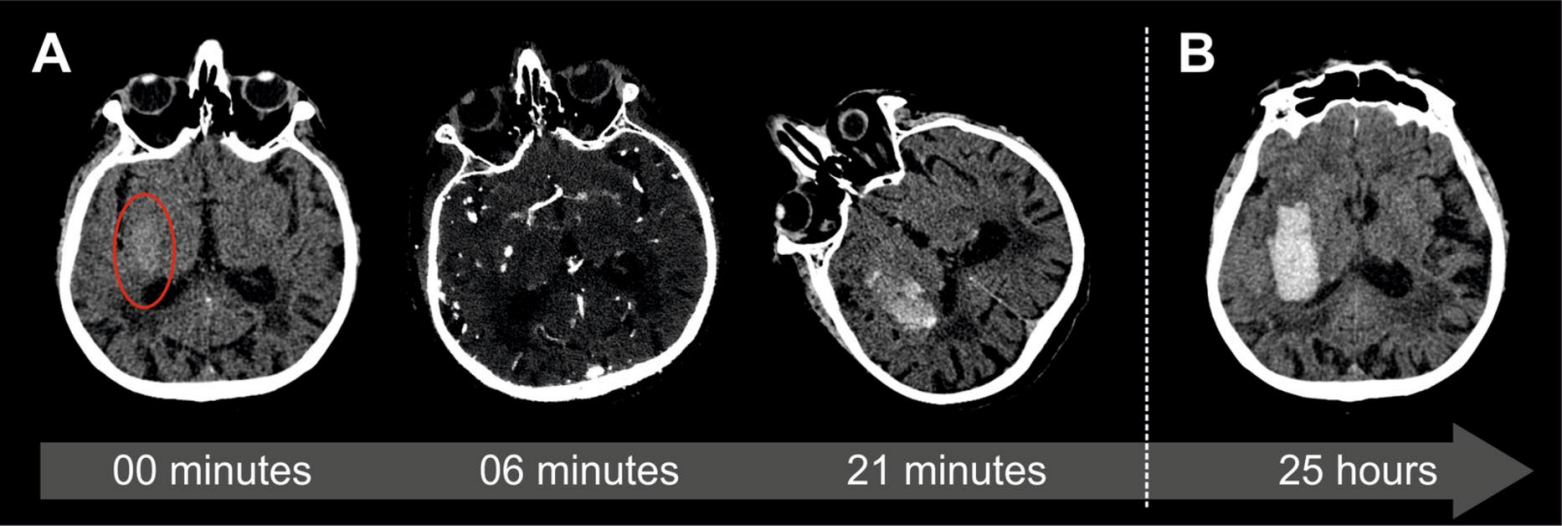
Hyperacute right putaminal hemorrhage on consecutive CT scans. A Hyperacute phase: non-contrast CT (0 min), CTA (6 min), and CT (21 min). B Acute phase: non-contrast CT (25 h). The red circle shows an area of slight hyperdensity representing fluid blood. Acute neurological deterioration demonstrated by progressive head and eye deviation (panel A) on image planes containing at least one of the lenses. The slice shown in panel B is 16 mm above the plane of the lenses
